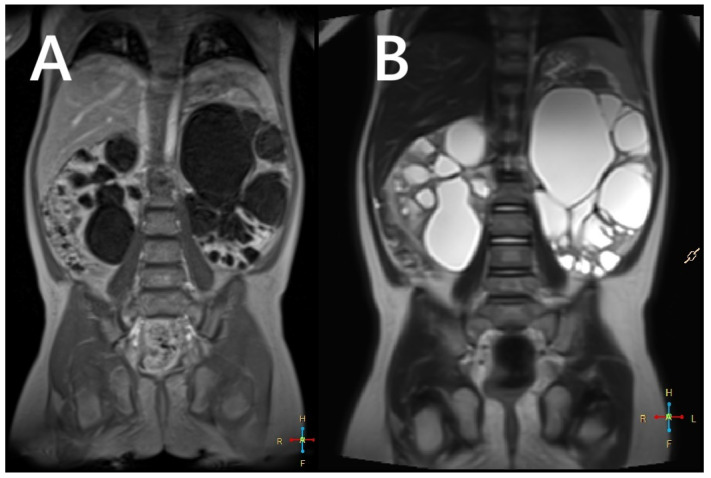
An MRI without (A) and with a contrasting agent (B) of a 3-year-old boy with a contiguous gene deletion syndrome involving TSC2/PKD1. Both kidneys are markedly enlarged, containing numerous thin-walled cysts of varying shapes. The remaining renal parenchyma is severely deformed.
